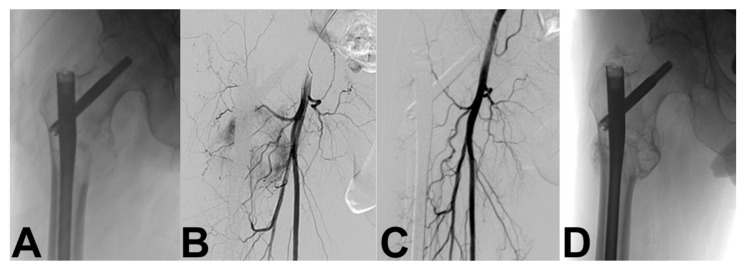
A 55-year-old man with right hip bone metastasis from renal cancer. (A) Prophylactic hip nail was done, (B) followed by digital subtraction angiography that showed the pathological tumor vessels, and (C) N-2-butyl cyano-acrylate selective embolization. (D) Post-embolization radiograph shows bone sclerosis (healing) of the bone metastasis without evidence of lesion progression at 6-month follow-up.
